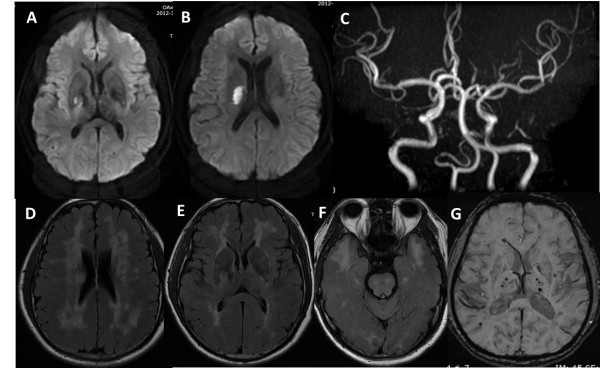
MRI findings. A-B. Diffusion-weighted imaging shows an acute cerebral infarct on the right basal ganglia and periventricular areas. C. MR angiography reveals no significant stenosis or malformation of major cerebral arteries. D-F. FLAIR image demonstrates hyperintensity lesions in the periventricular white matter, basal ganglia, and brainstem, characteristically in the external capsule and anterior temporal pole. G. Susceptibility weighted imaging shows multiple cerebral microbleeds in the bilateral basal ganglia.
